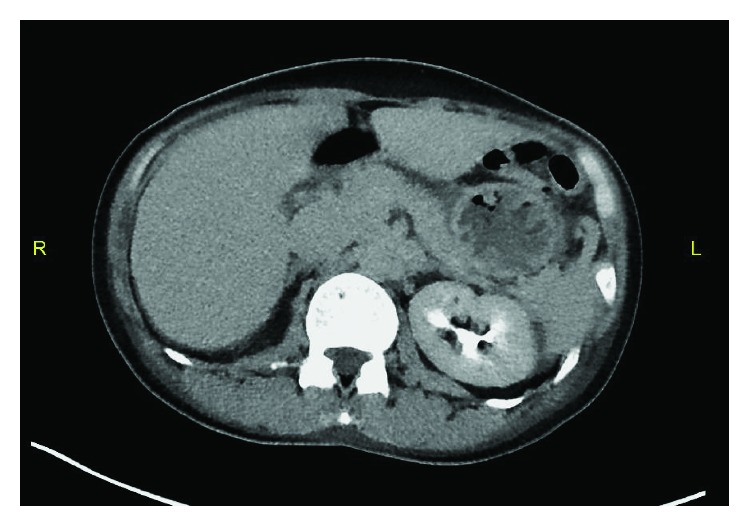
Repeat CT scan showing the absence of the previous liver artifacts.
